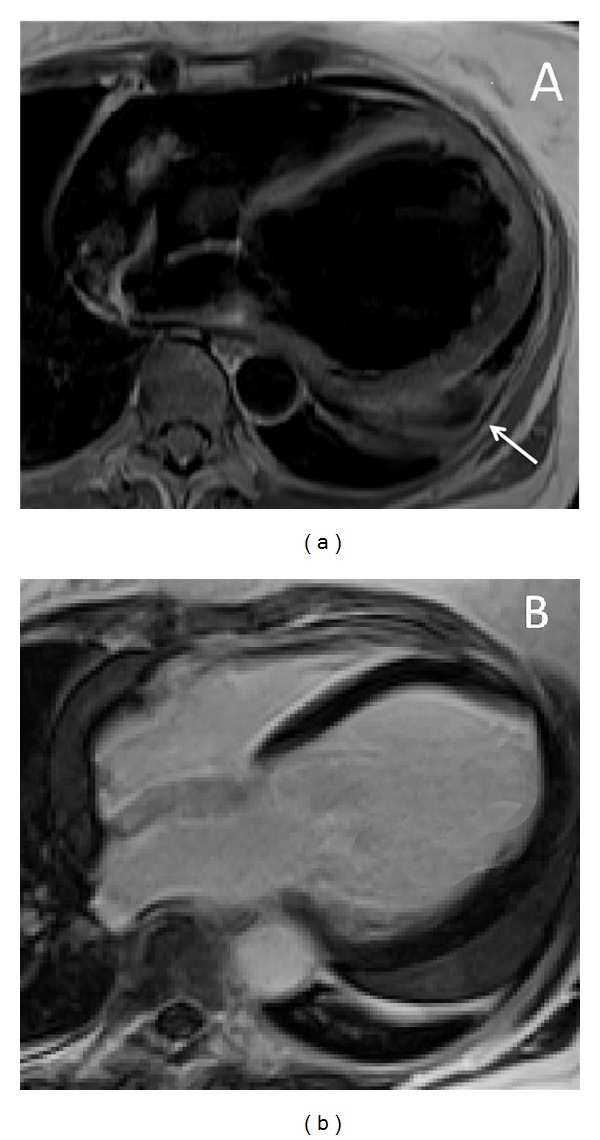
Idiopathic dilated cardiomiopathy in a 27-year-old asymptomatic patient occasionally identified during a routinary clinical screening. T1-weighted turbo spin echo 4-chamber image (a) shows a hugely dilated left ventricular chamber (end-diastolic volume 296 mL; ejection fraction 26%). Late gadolinium enhancement image acquired on the same orientation plane (b) shows absence of pathological enhancement within both ventricular chambers. There is also concomitant significant amount of pericardial effusion (arrow) located within the midbasal left ventricular lateral free wall which was initially interpreted as expression of a post pericardiomyocarditis form.
